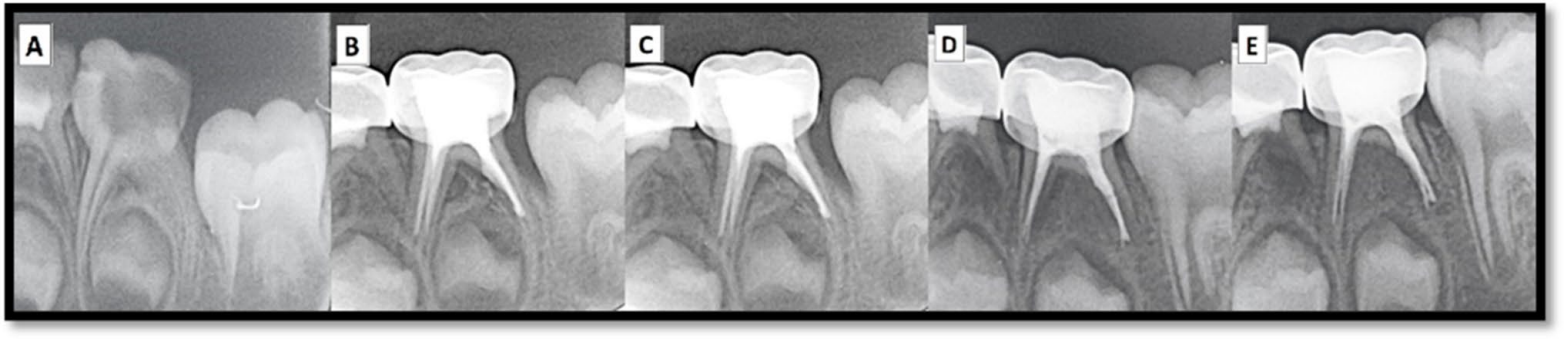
A Preoperative intraoral peri-apical(IOPA) radiograph of lower left second primary molar. B immediate IOPA radiograph post-obturation with zinc oxide-olive oil paste. C–E Post-IOPA radiographs at 3, 6, and 12 months
